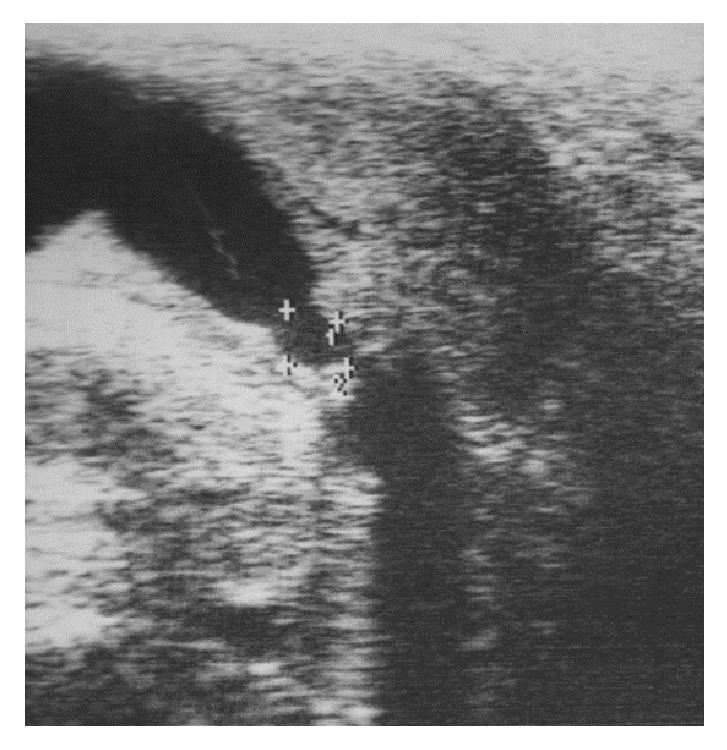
Ultrasound of the bulbar urethra.* Ultrasound shows an irregular, hyperechogenic zone in the bulbar urethra, representing a bulbar urethral stricture*.
